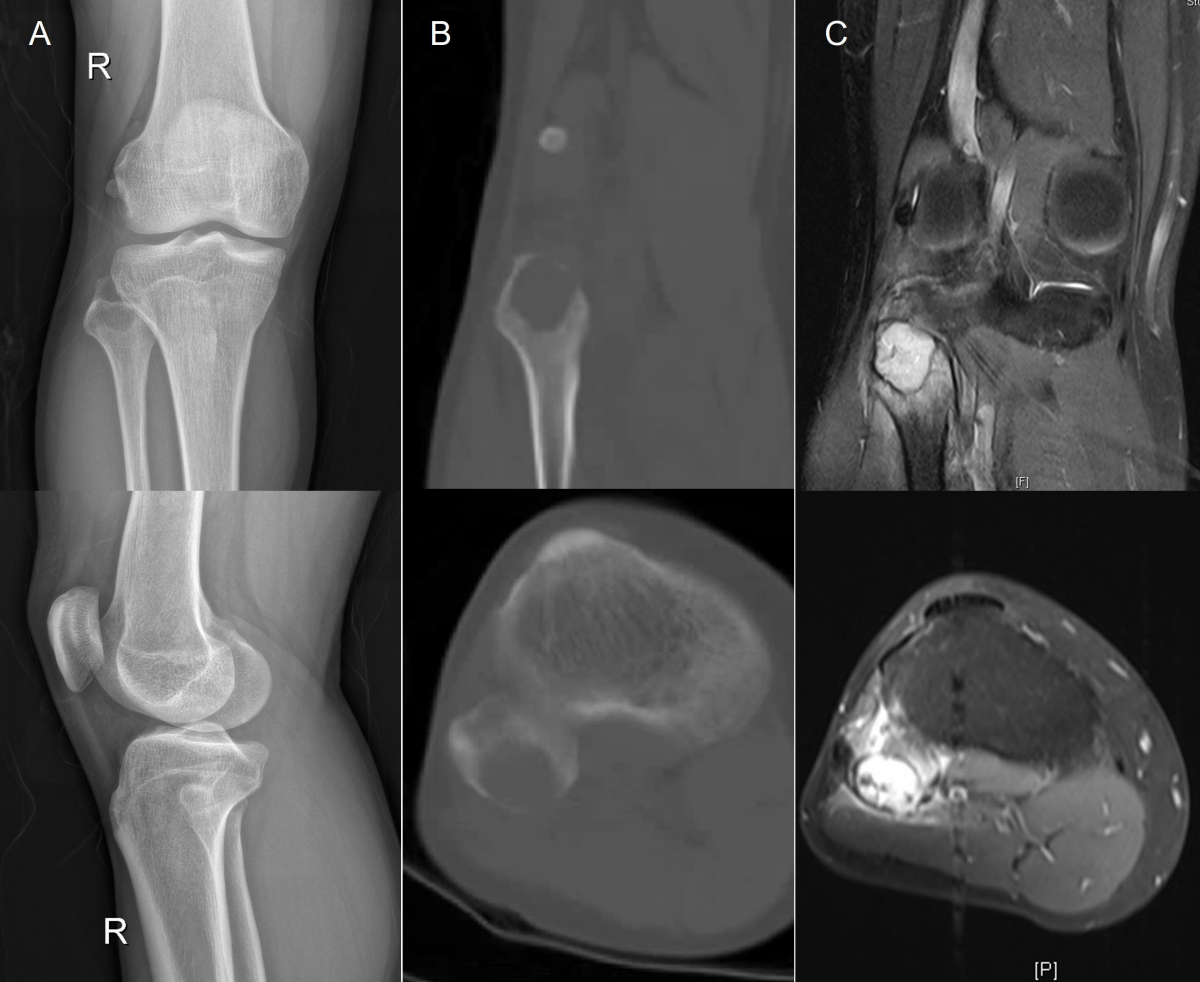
(A) X-ray of the right knee joint revealed a rounded, expansive bony destruction of the right fibular head, measuring (23mm×27mm). (B, C) CT scan and MRI revealed bony destruction of the right fibular head accompanied by a posterior soft tissue mass, measuring (16mm×24mm), suggestive of a neoplastic lesion. CT, computed tomography; MRI, magnetic resonance imaging.
